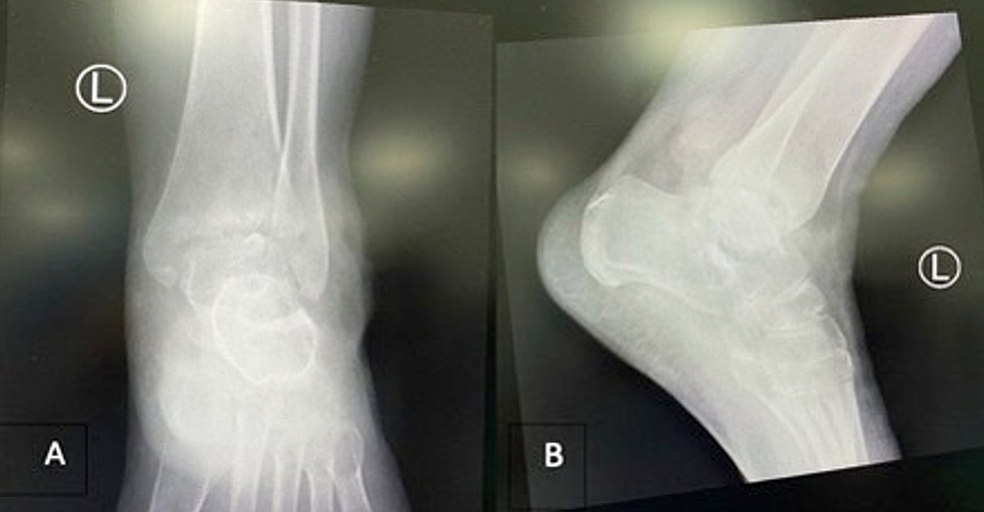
(A) Anteroposterior and (B) lateral plain radiographs showing mild arthritic changes and soft tissue swelling
